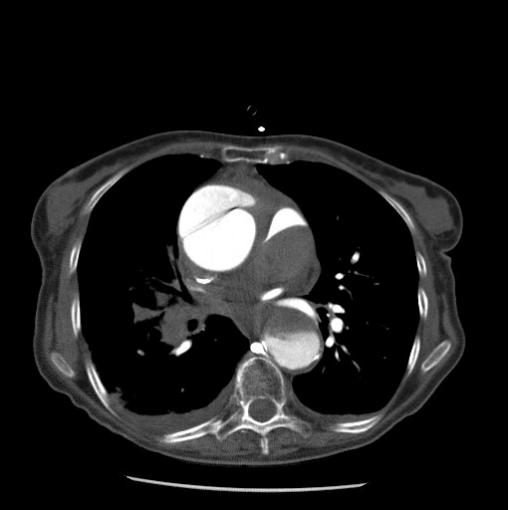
CT image of occluded right pulmonary artery and aortic dissection. Contrast enhanced CT image at the level of the right pulmonary artery demonstrates an aneurismal ascending aorta (AA) with a dissection flap and adjacent mediastinal haematoma. The right main pulmonary artery (RPA) is occluded.
